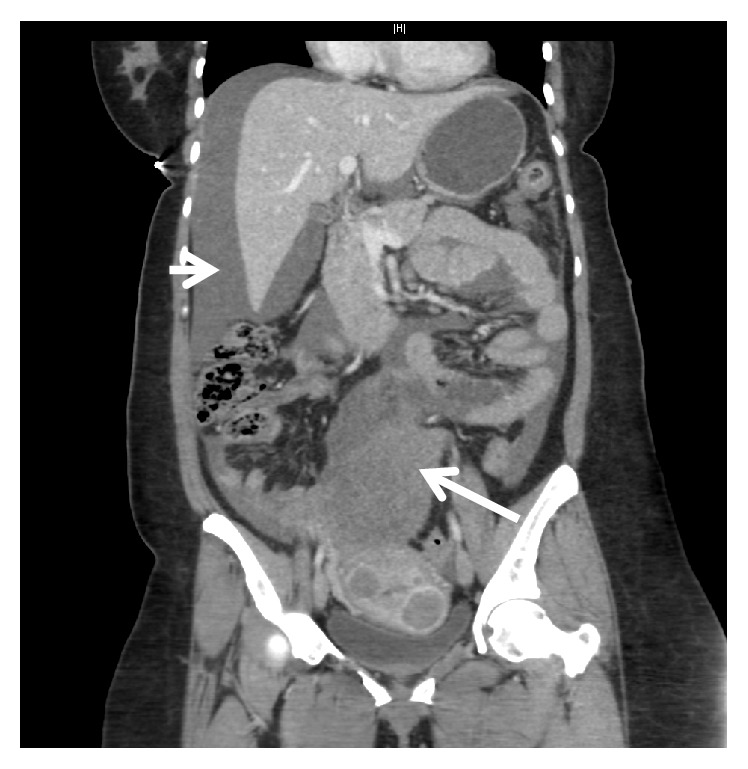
Computed tomography of the abdomen and pelvis showing the 8.8 × 7.3 × 8.3 cm degenerated fibroid (arrow) and free fluid in Morison's pouch (arrowhead).
